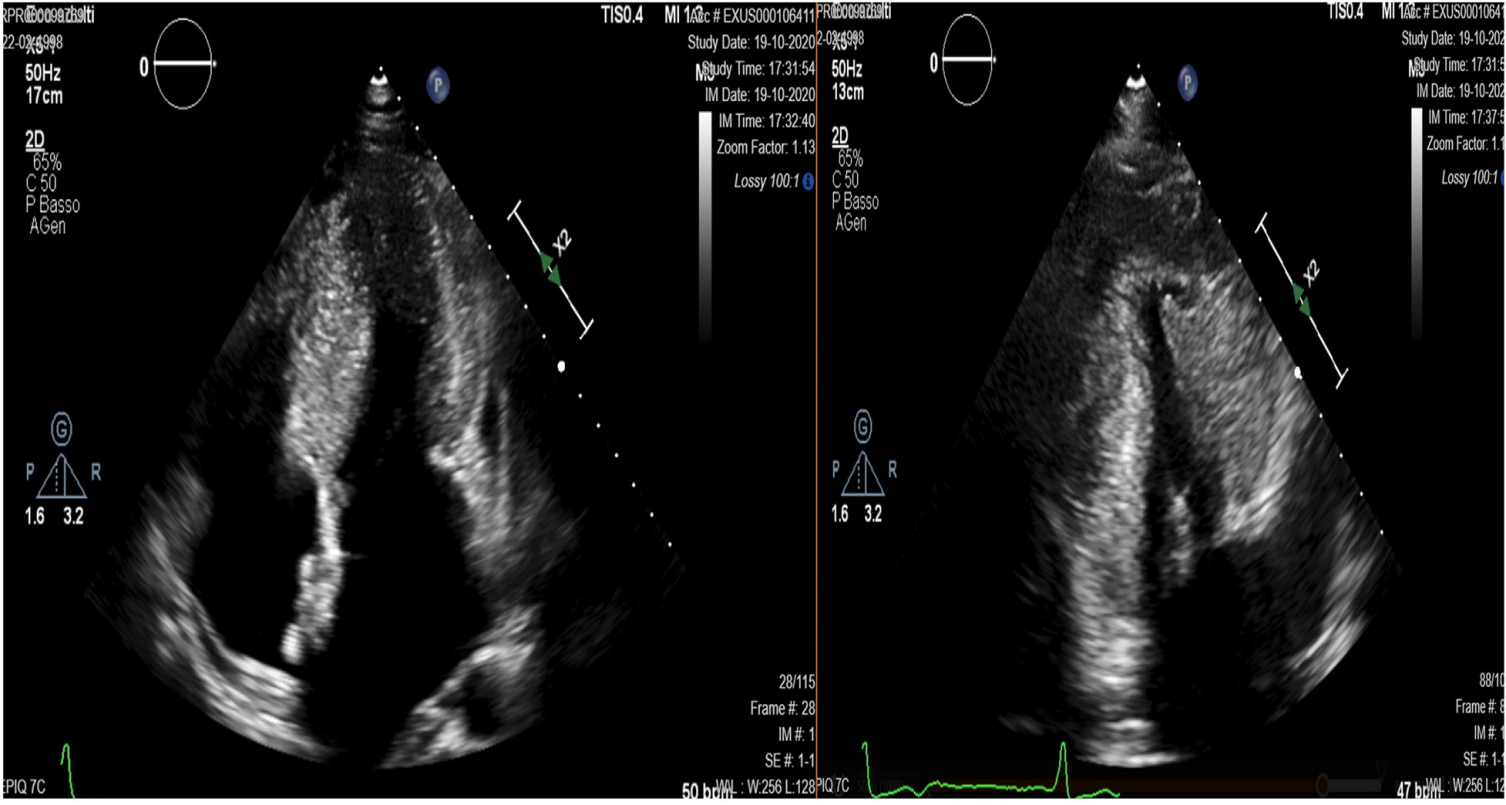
Ultrasound scan shows a concentric left ventricular parietal hypertrophy associated with a 23 mm mid- basal interventricular septum thickness.
